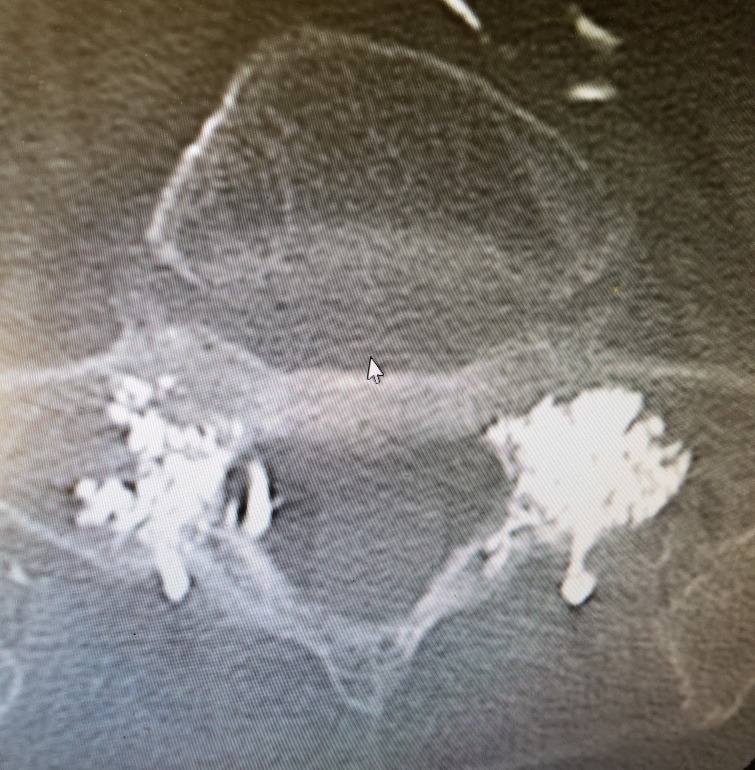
Postoperative axial CT of sacral alae obtained after bilateral sacroplasty demonstrating anteromedial spread of Cortoss filling in associated fracture sites.
